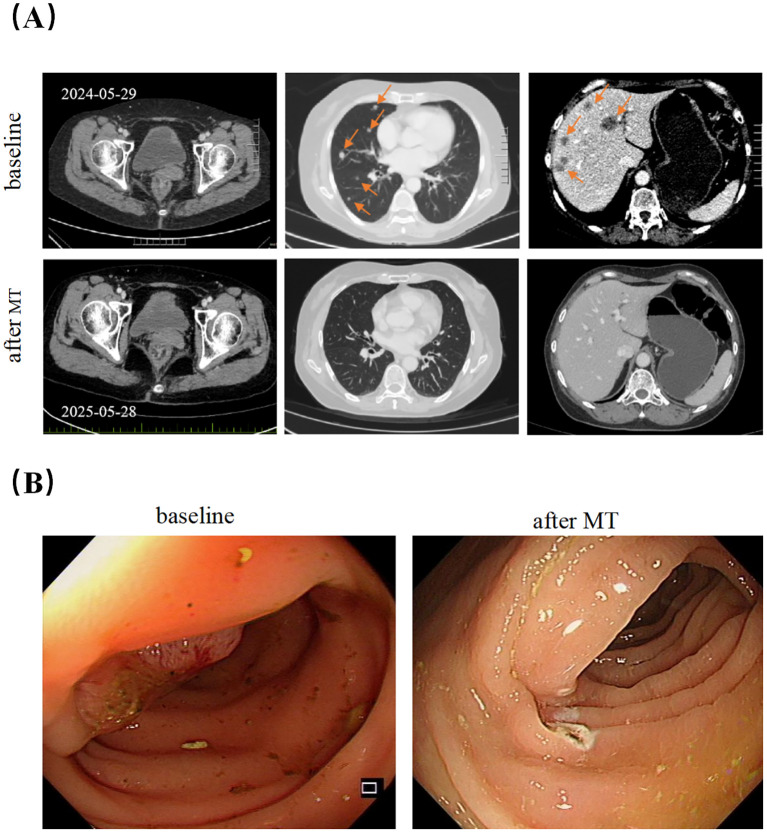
Radiographic and endoscopic comparison of primary and metastatic lesions before and after maintenance therapy. (A) CT imaging changes of primary lesions, liver and lung metastases before treatment and after maintenance therapy. (B) Comparison of endoscopic images before and after maintenance therapy.
